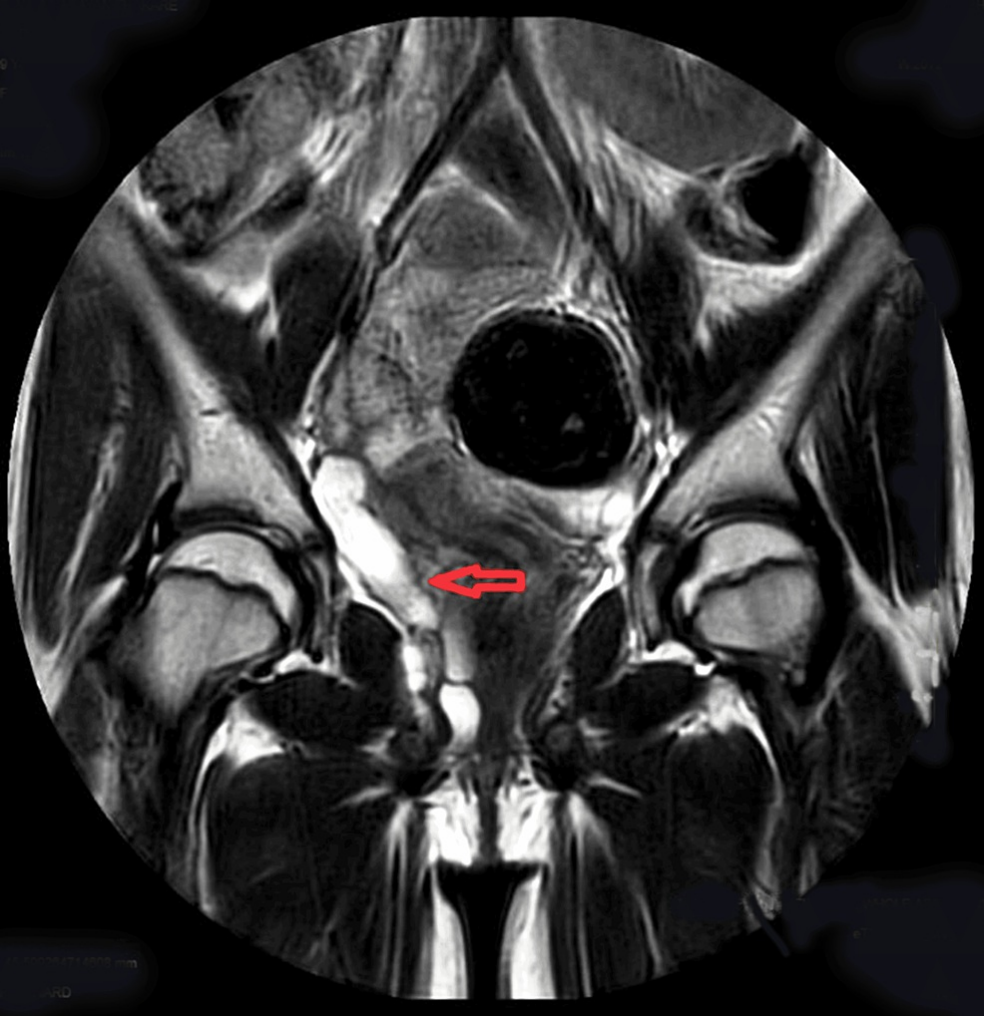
MRI showing moderate to gross right-sided hydroureter; it is tortuous in course with ectopic insertion in urethra near external urethral orifice
